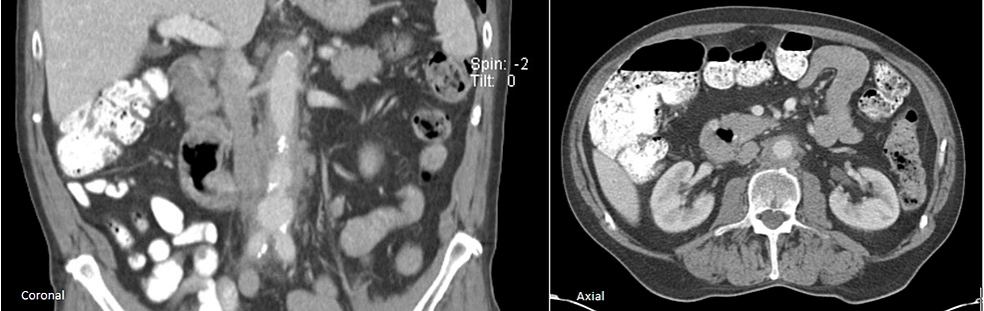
Initial CT abdomen Coronal and axial planes demonstrate a moderate degree of circumferential thickening of the periaortic space including the aortic wall. This inflammation started at the inferior thoracic aorta and extended past the aortic bifurcation till the middle of the right iliac artery CT: computed tomography
